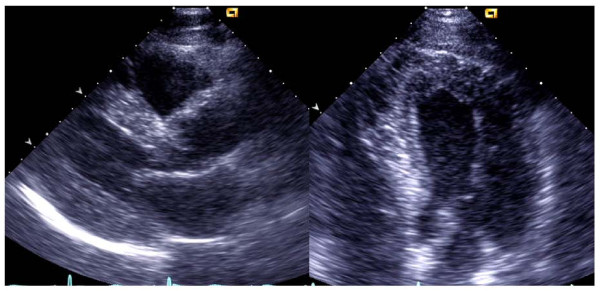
Echocardiography demonstrating concentric left ventricular hypertrophy in parasternal long-axis and apical 4-chamber view.
